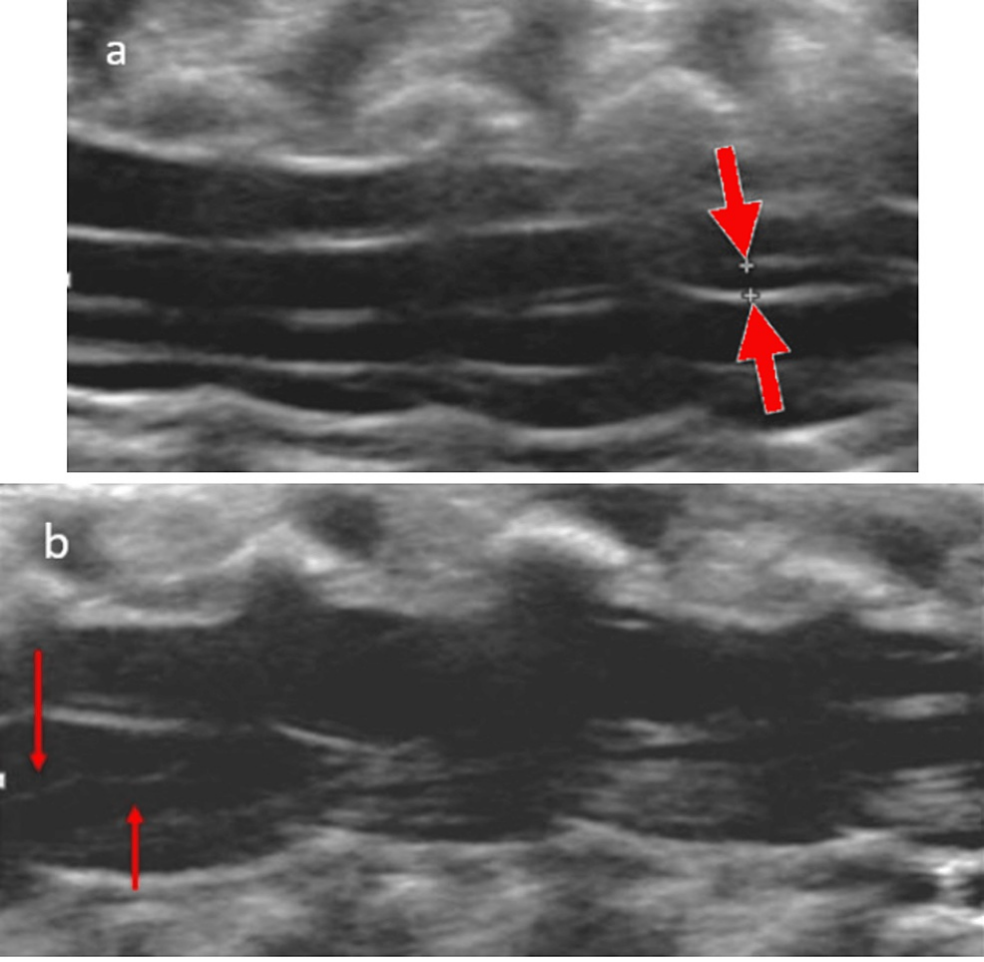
Ventriculus terminalis (a) Longitudinal ultrasound image demonstrating cystic dilatation within the conus medullaris continuous with the central canal (red arrows). (b) Follow-up ultrasound at 6 weeks of age demonstrates resolution Radiological images obtained by the authors
